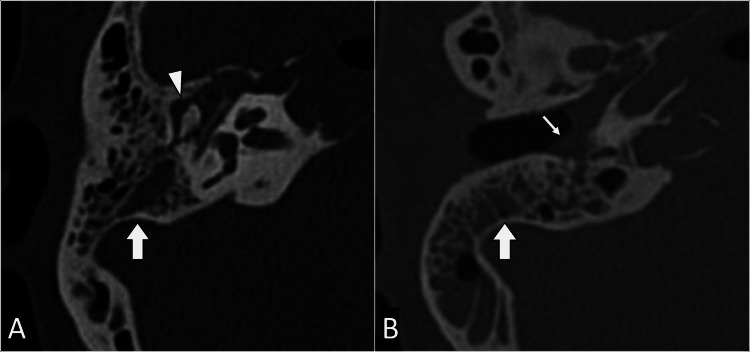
Axial CT images of the right internal acoustic meatus (IAM) in bone (A) and soft tissue (B) windows reveal soft tissue material within the external auditory canal (arrow), accompanied by complete opacification of the mastoid air cells (block arrow) and the presence of middle ear effusion (arrowhead). CT: Computed tomography
